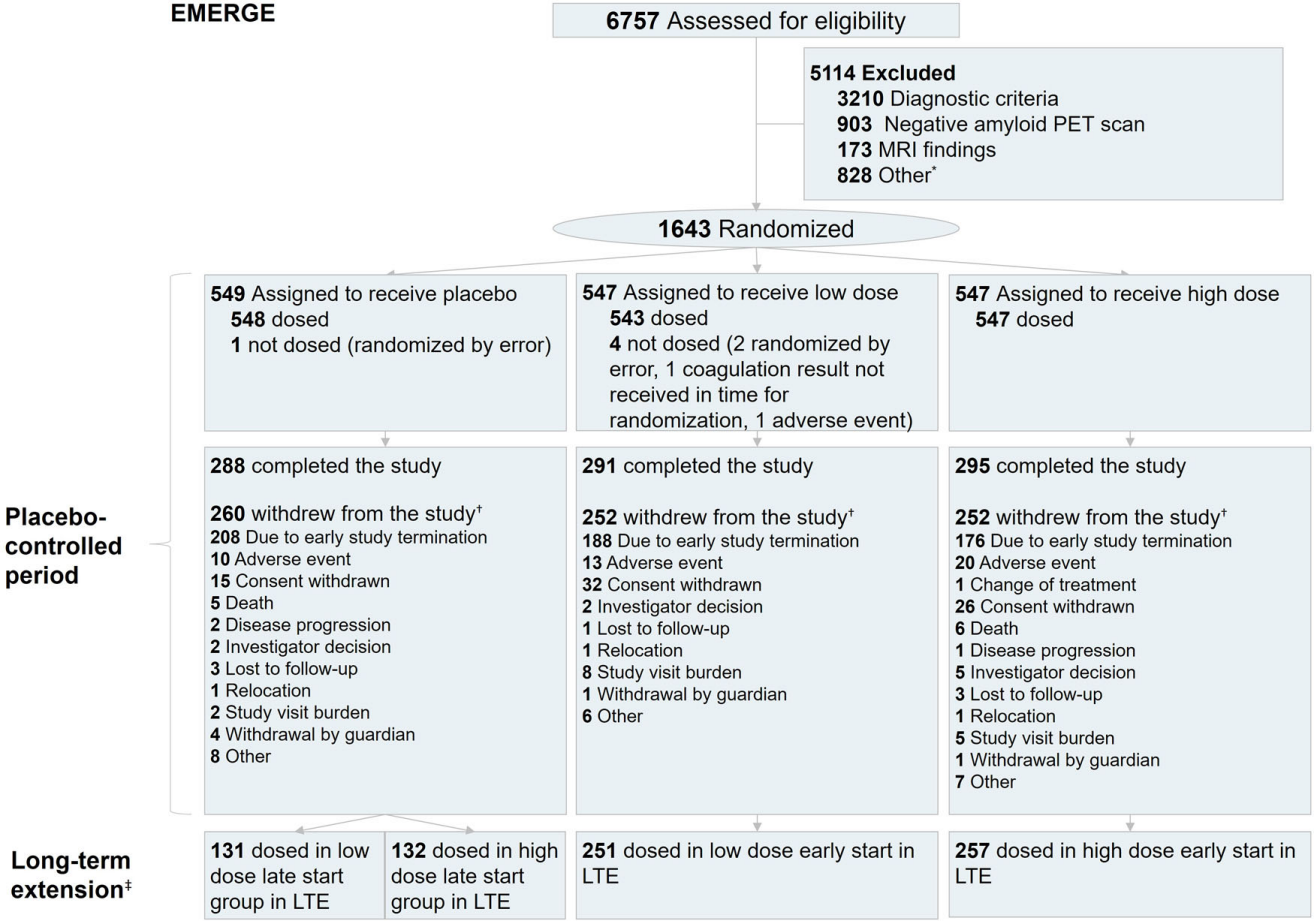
Trial profile. *Other reasons for not meeting inclusion/exclusion criteria include inability to comply with study requirements; presence of diabetes mellitus that, in the judgment of the investigator, cannot be controlled or adequately managed; inability to understand the purpose and risks of the study and provide signed and dated informed consent and authorization to use protected health information in accordance with national and local subject privacy regulations; other unspecified reasons that, in the opinion of the investigator or sponsor, make the individual unsuitable for enrollment; history of or positive test result at screening for hepatitis C virus antibody or hepatitis B virus (defined as positive for both hepatitis B surface antigen AND hepatitis B core antibody); use of allowed chronic medications at doses that have not been stable for at least 4 weeks prior to screening visit 1 and screening up to day 1, or use of Alzheimer's disease (AD) medications at doses that have not been stable for at least 8 weeks. ^†^Some categories with < 1% patients are not displayed, including loss of capacity, pregnancy, and protocol amendment. ^‡^Following the 78‐week placebo‐controlled study period, there was an optional dose‐blind long‐term extension (LTE) period up to week 134. MRI, magnetic resonance imaging; PET, positron emission tomography.
